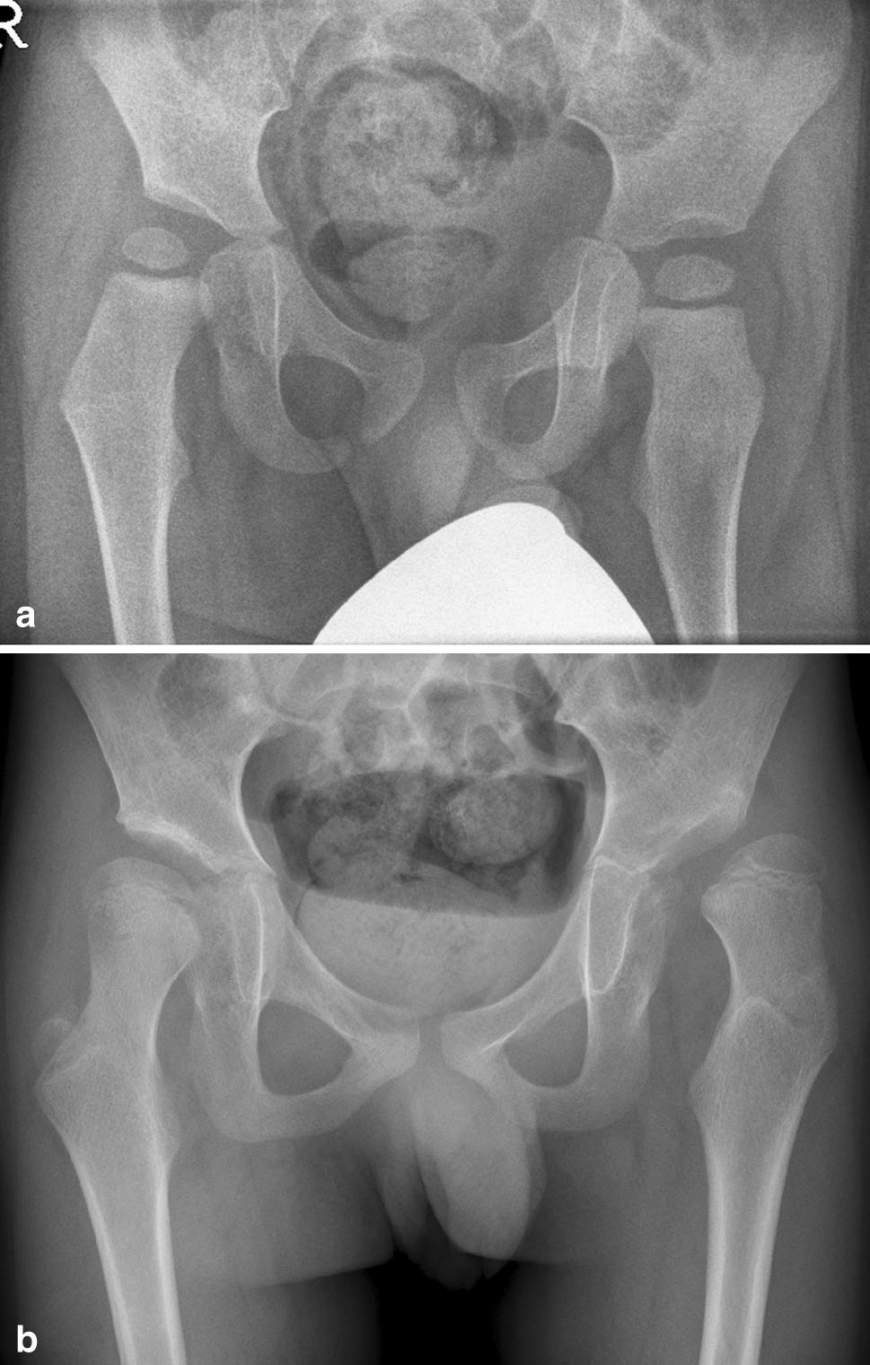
AP radiographs of a boy with GMFCS level V cerebral palsy. a At T1 (22 months of age), the patient had a head-shaft angle (HSA) of 169° right and 185° left, with a migration percentage (MP) of 29 % right and 0 % left. b At T3 (95 months of age), the right hip was displaced laterally with a MP of 34 % and the left hip with a MP of 76 %
